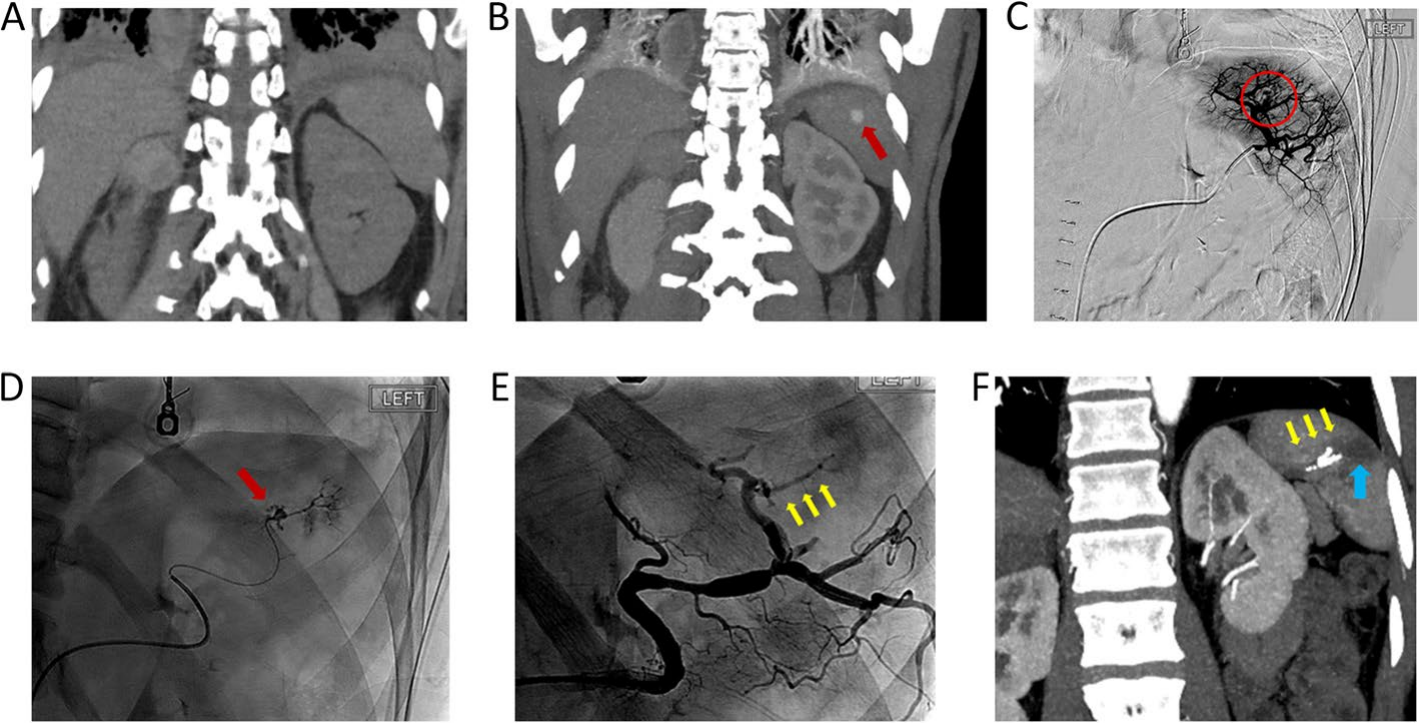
A and B Contrast computed tomography demonstrated a traumatic splenic artery pseudoaneurysm (A pre-contrast image; B arterial phase maximum intensity projection) C and D) Catheter angiography via the splenic artery (C) and segmental artery (D) demonstrate pseudoaneurysm (red circle and red arrow). E Post-embolization angiogram suggested successful occlusion of the culprit artery. Yellow arrow: Obsidio embolic. F Follow-up computed tomography demonstrated splenic infarct in the embolization territory (blue arrow) of the embolized territory. Yellow arrow: Obsidio embolic
